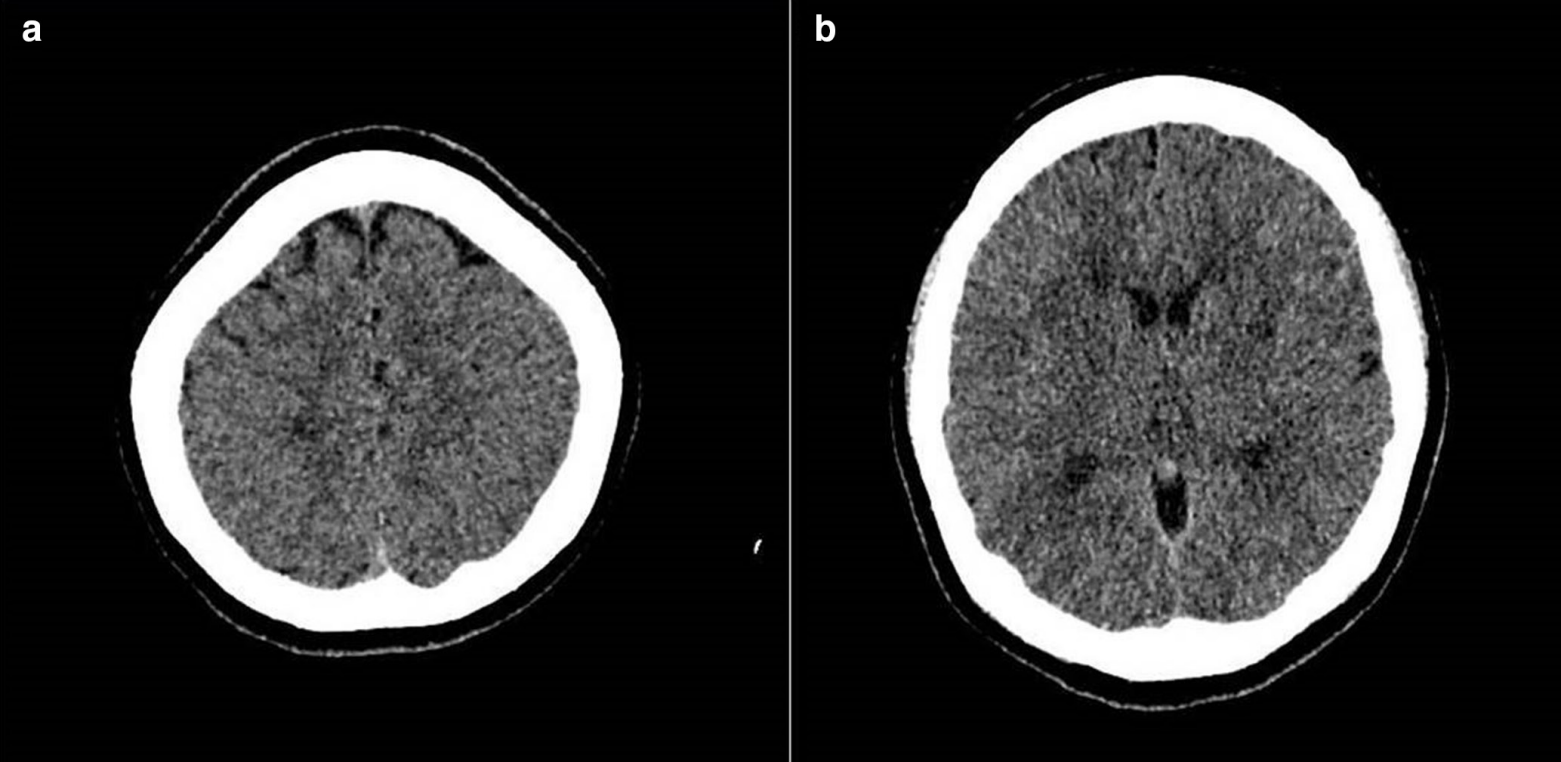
Axial non-contrast CT brain A and B demonstrate multiple hypodense lesions in bilateral cerebral hemispheres.
